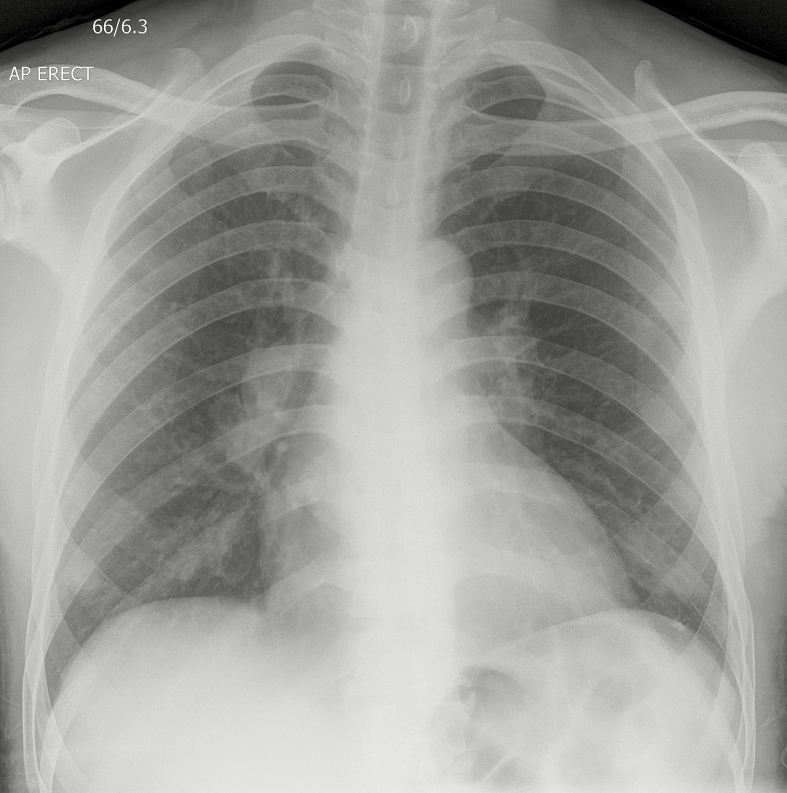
Chest radiograph with bilateral hilar lymphadenopathy and bilateral nodular pulmonary infiltrates.
